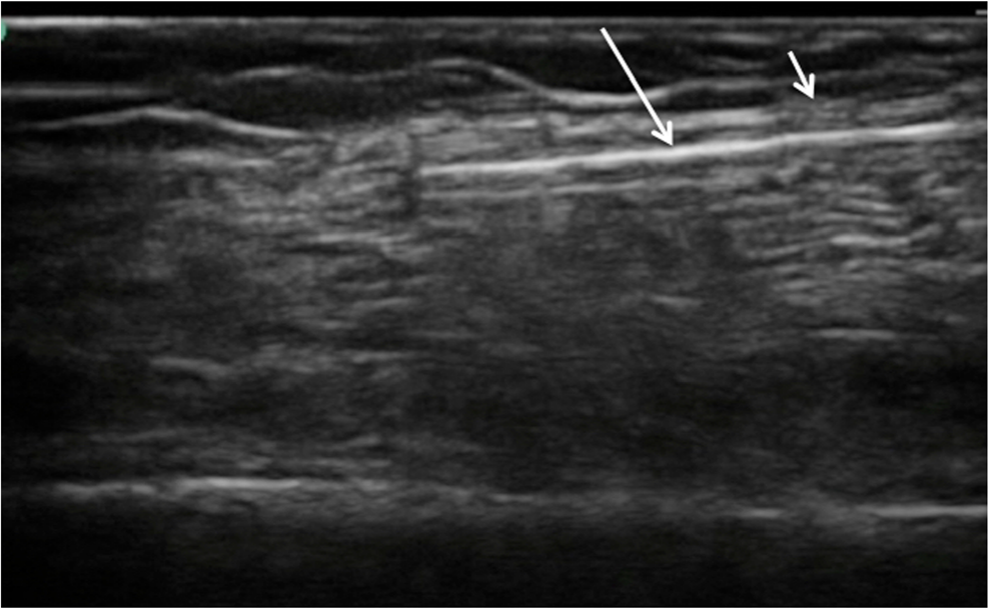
Real-time ultrasound image of blunt tunneling (*long white arrow*) device passing immediately deep to fascial plain (*short white arrow*)
